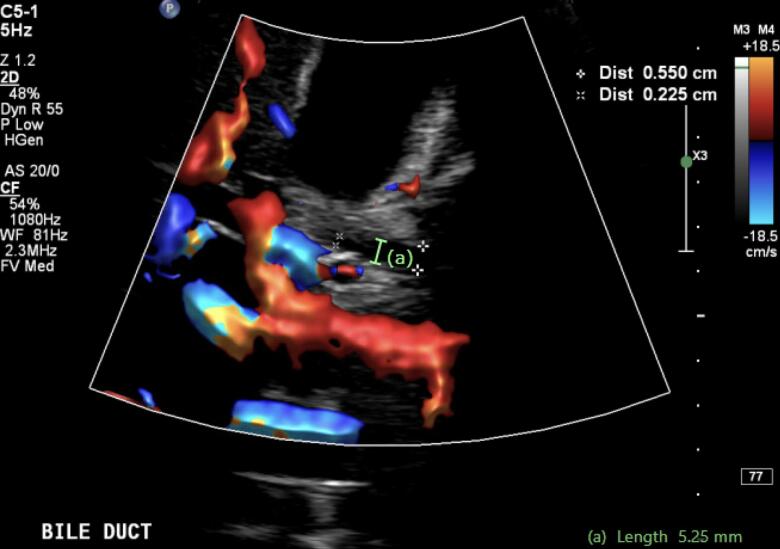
Initial ultrasound demonstrating non dilated proximal common bile duct. Limited view due to patient's body habitus.
